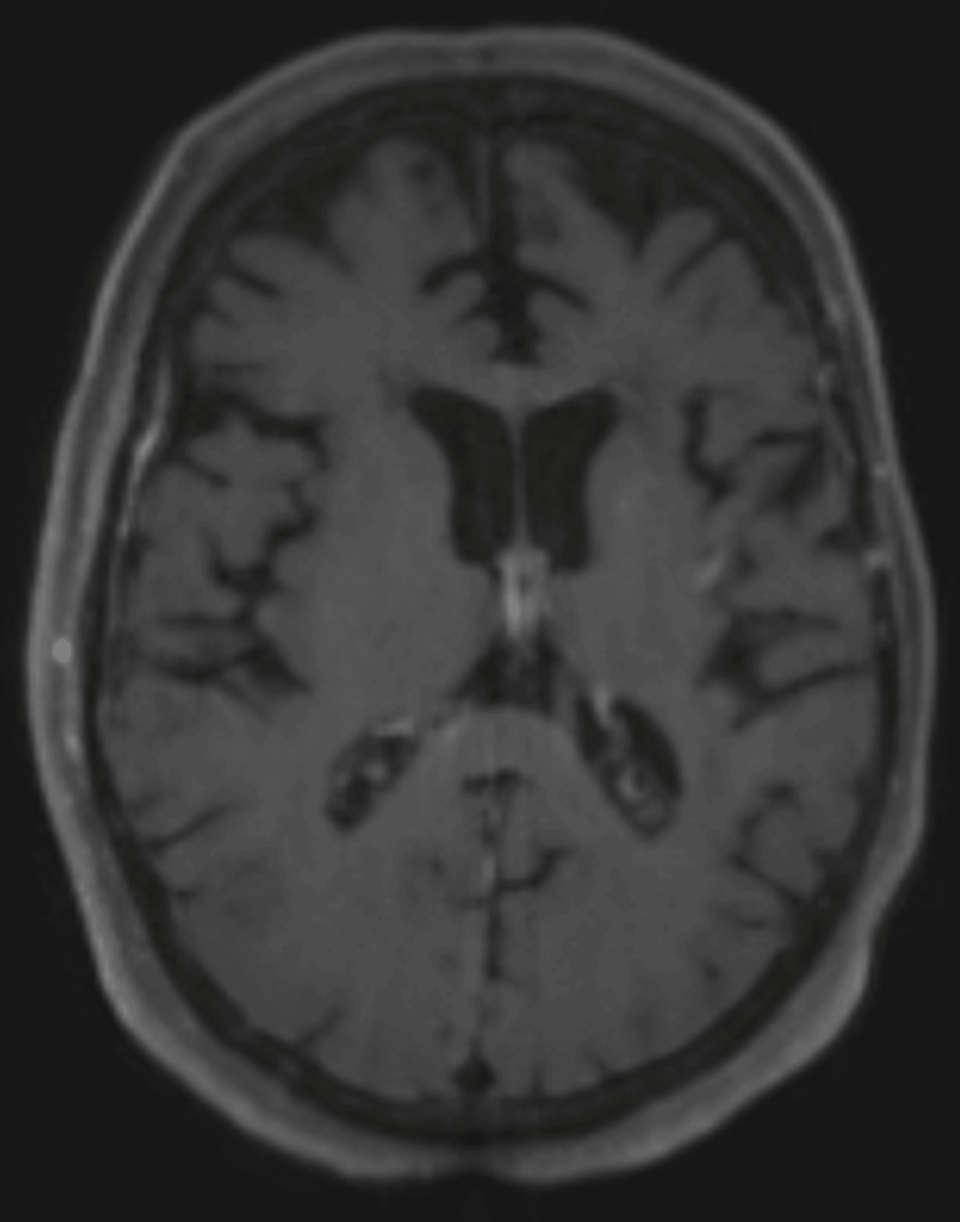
10-year post-operative MRI (T1 with gadolinium contrast) Complete resection without evidence of any lesion.
